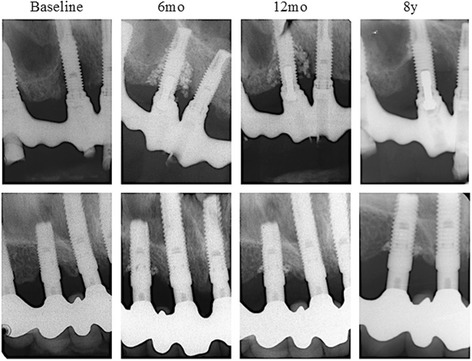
PTG observed in radiographs at the 8-year follow-up
